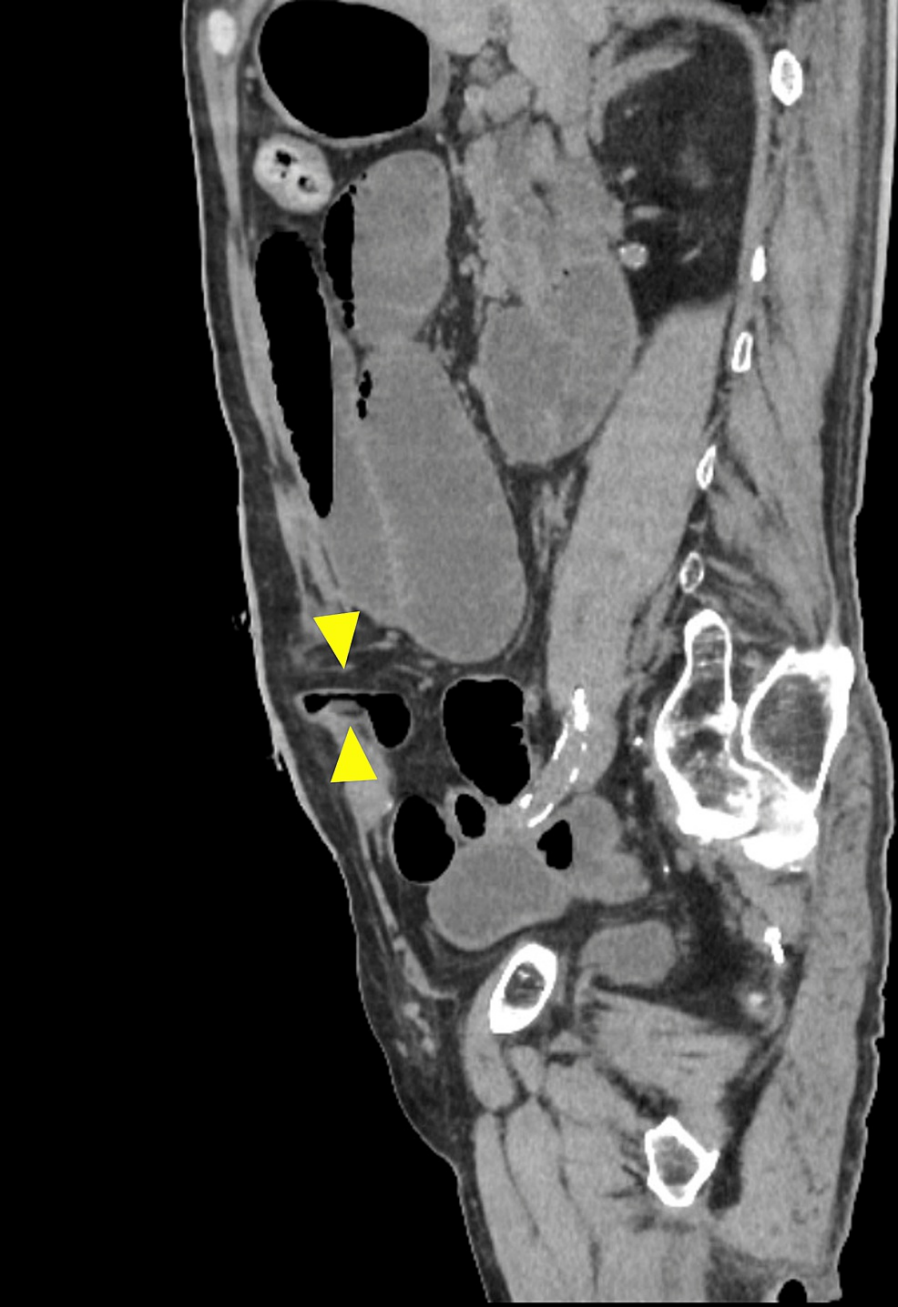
CT image of a small bowel obstruction at the stoma site (sagittal view) The arrowheads indicate a luminal narrowing of the stoma limb causing small bowel obstruction. Image created by the authors
